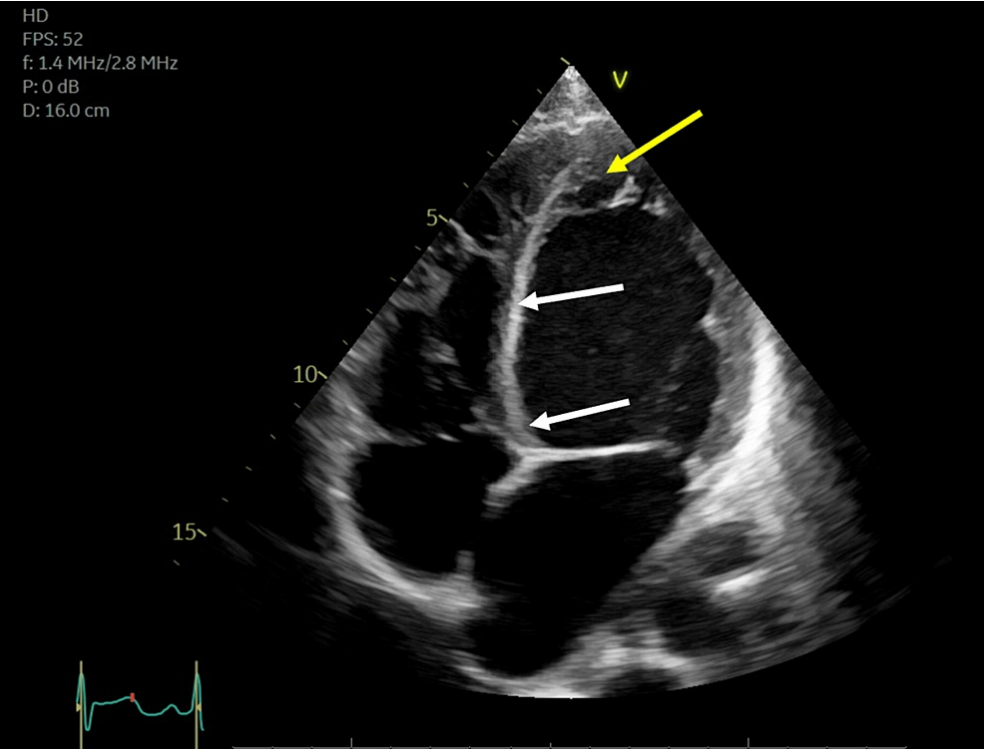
Echocardiogram in ventricular systole The yellow arrow indicates left ventricular apical walls are normokinetic as evidenced by normal thickening and endocardial excursion (compared to Figure 3). White arrows indicate left ventricular mid-segment and basilar walls are akinetic as indicated by the absence of wall thickening and endocardial excursion.
